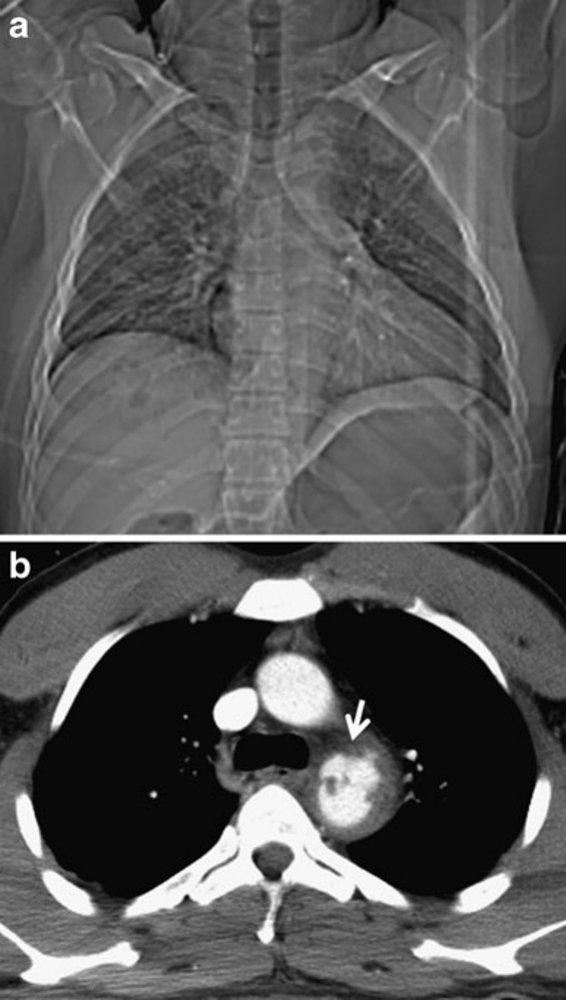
a, bPatient with laceration of the aorta. aTopogram (analogical to chest radiograph) shows normal mediastinal width. bMDCT reveals rupture of the aortic isthmus with small periaortic hematoma (*arrow*)
